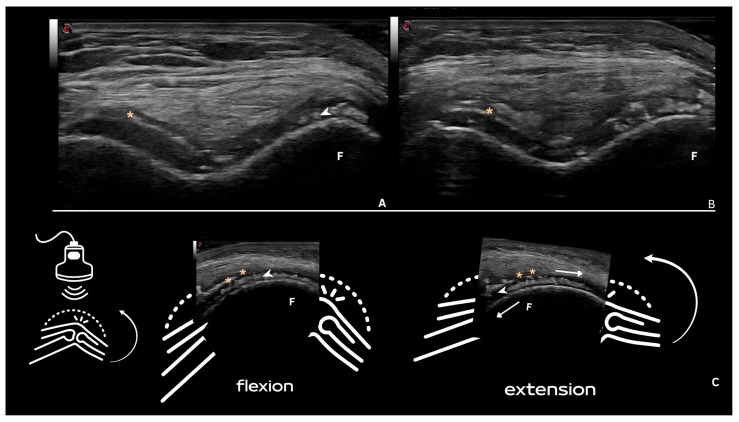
Pseudo-double contour. (A,B)—Femoral intercondylar transverse ultrasound scan with hyperechoic deposits within the hyaline cartilage parallel to the surface of the cartilage, without posterior shadowing (arrowhead). (*) Pseudo-double contour sign. F—Femoral condyle. (C)—Behavior during dynamic scanning (i.e., joint movement): The deposits within the cartilage remain fixed and move together with the femoral condyle (arrowhead), and the pseudo-double contour sign moves in the opposite direction (located at the chondro-synovial interface or in the joint capsule and adjacent ligaments. Source: personal files.
